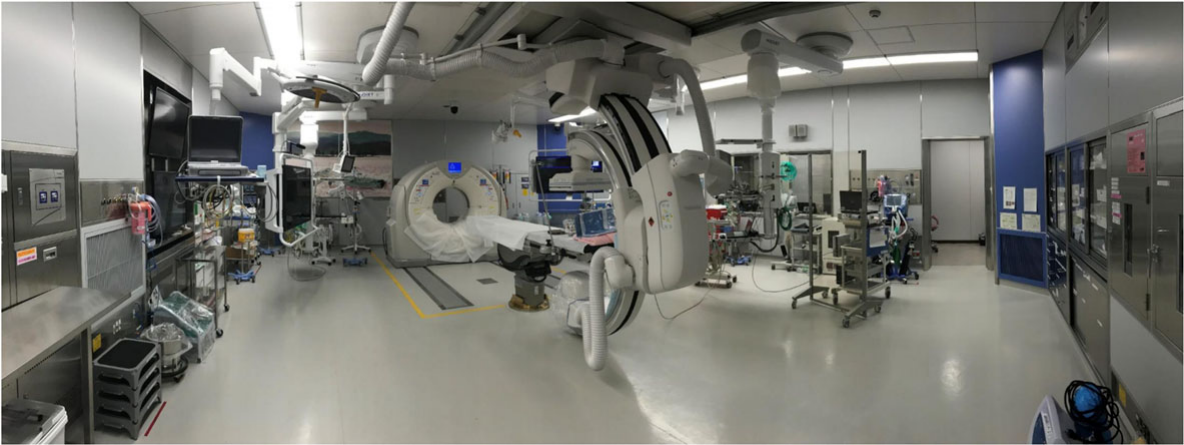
A photograph of rotated type hybrid ER system (Canon Medical Systems Corp., Ohtawara, Japan). C-arm is located on the wall at the opposite side of the CT gantry; therefore, this system can secure wide working space in resuscitation phase
